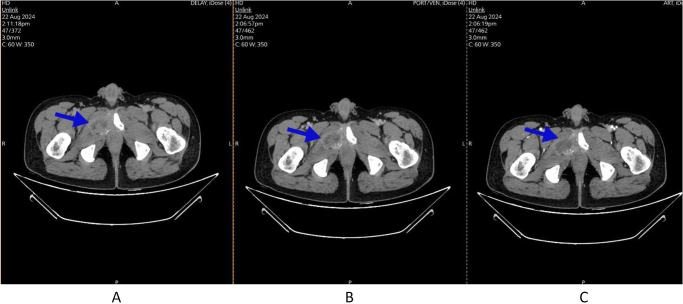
Axial views of CT scan with IV contrast for pelvic region was done and showed the following: a lytic lesion in right inferior pubic bone showed soft tissue component, with heterogeneous progressive enhancement post contrast administration (blue arrows): (A) delayed phase, (B) venous phase, and (C) arterial phase. A core needle biopsy was obtained from this lesion.
